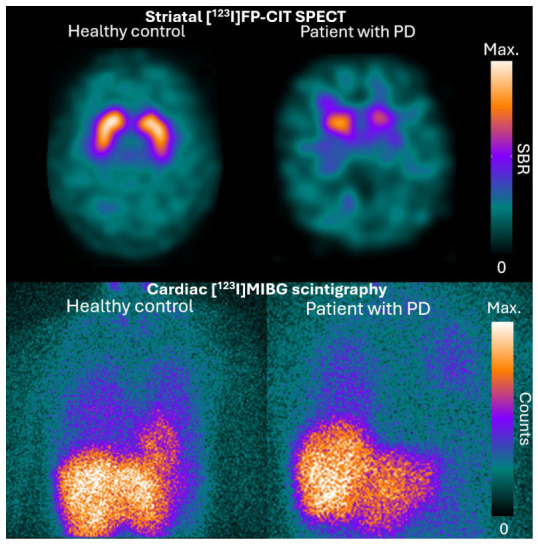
Typical examples of striatal [^123^I]FP-CIT SPECT and cardiac [^123^I]MIBG scintigraphy. SBR = Specific Binding Ratio.
